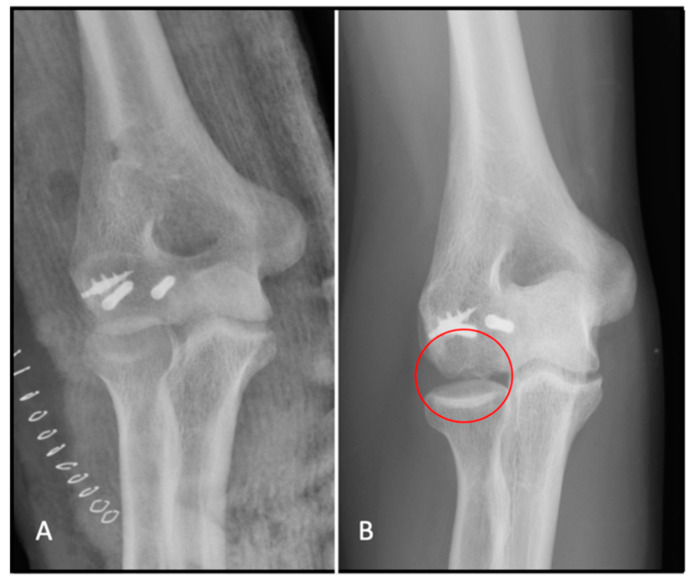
(A) Post-operative X-ray; and (B) signs of avascular necrosis circled on the follow-up X-ray.
